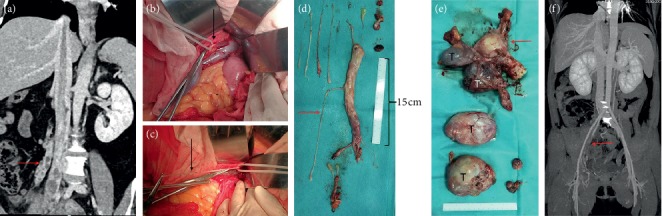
Second-stage surgery for case 5. (a) The tumor enters the inferior vena cava from the right iliac vein and the right ovarian vein (arrow). (b) The right ovarian vein is visible through the venous vein wall. (c) The tumor is pulled out from the right ovarian vein. (d) Tumors in the inferior vena cava/iliac vein/ovarian vein. (e) Pelvic tumor. Arrow refers to the uterus and bilateral attachment; (f) CT scan at 6 months after surgery shows unobstructed inferior vena cava and thrombosis of right iliac vein (arrow).
